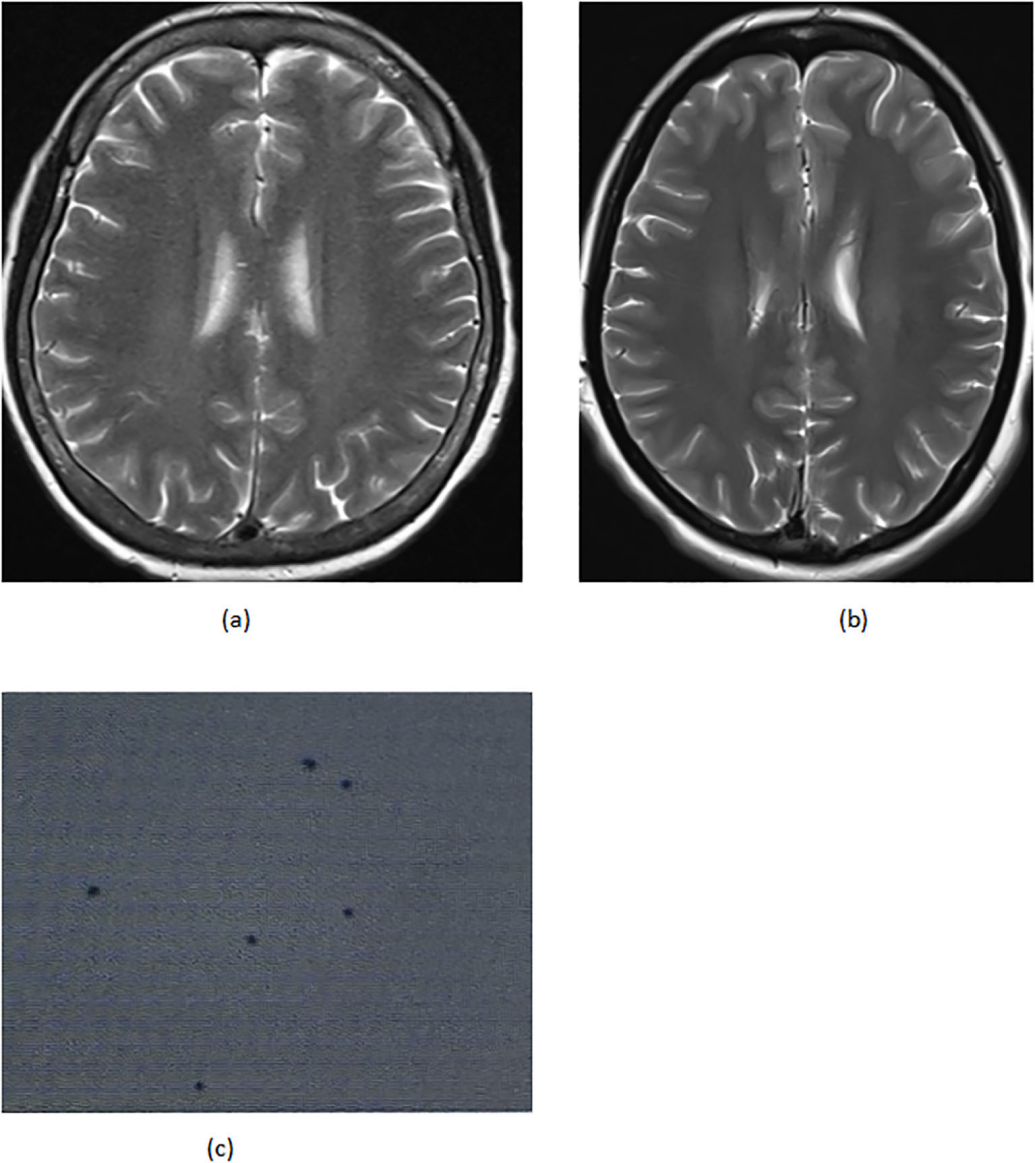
(A) 05–07 the brain MRI (B) 06–03 the brain MRI (C) 05–14 cerebrospinal fluid biopsy.
